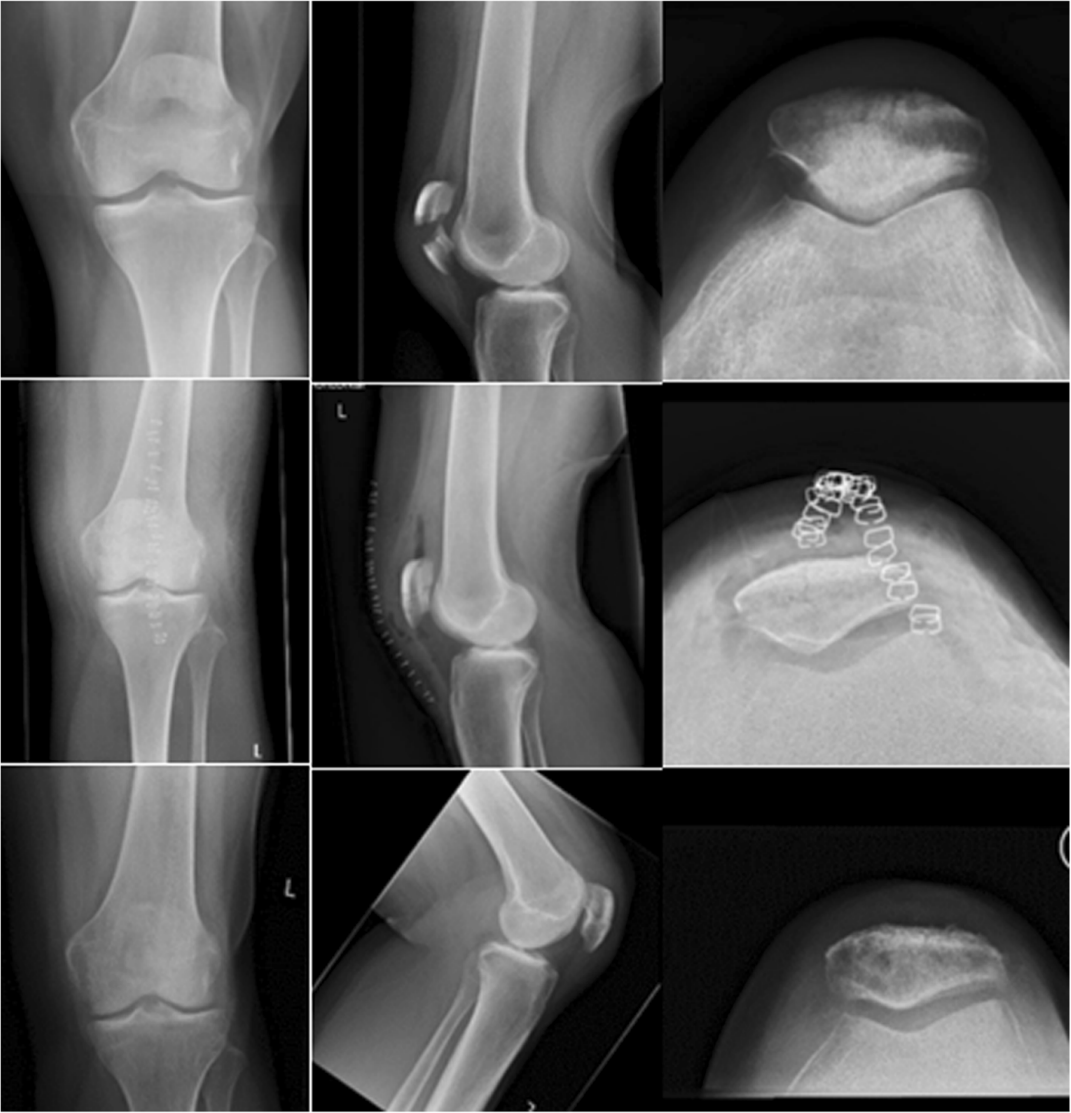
Pre-, post-, and 6-month postoperative X-rays (above, middle and below, respectively) of patient 6, a 58-year-old woman with a simple transverse patella fracture
